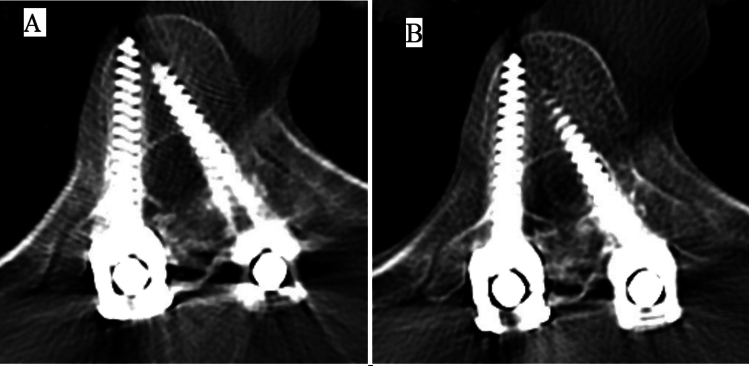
A Axial CT without contrast showing the left T8 screw breach into the spinal canal. B Axial CT myelogram at the level of T8 showing compression of thecal sac and cord by the medially misplaced screw
